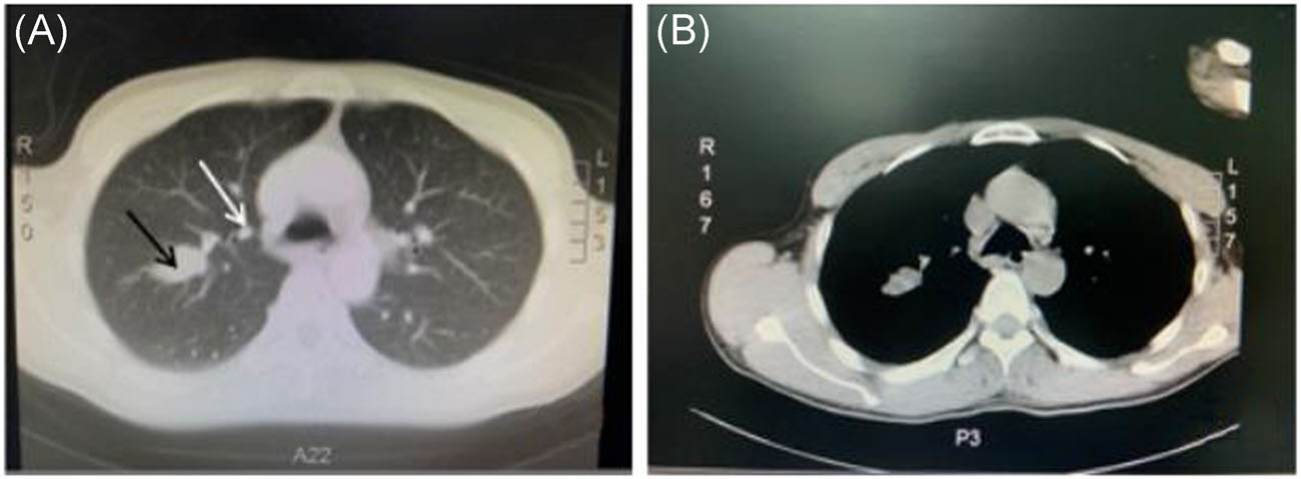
Chest CT examination. Irregular mass in the right upper lobe, about 27 mm × 18 mm (A, shown in black arrow), right hilar soft tissue mass (A, shown in white arrow), enhanced scan showed irregular soft tissue density shadow in the right hilar region, with uniform density on enhanced scan (B, shown in white arrow). CT, computed tomography [Color figure can be viewed at wileyonlinelibrary.com]
